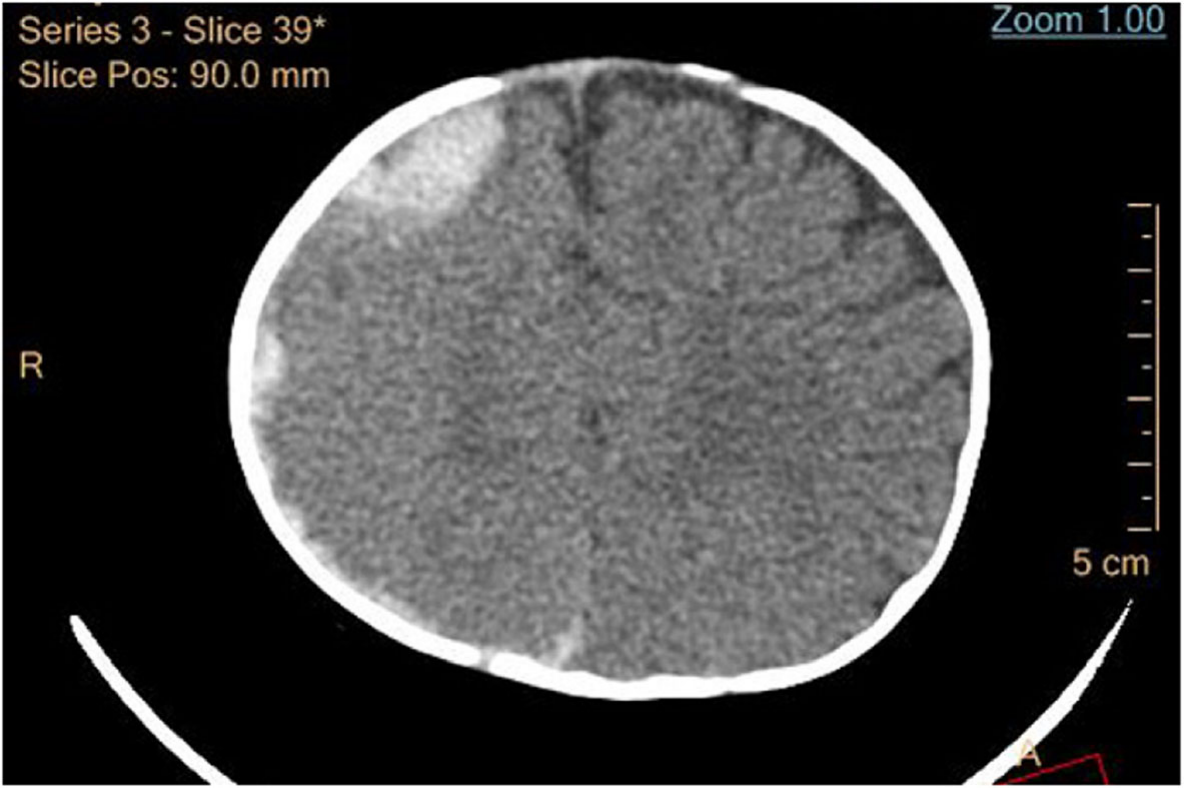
Three months after initial presentation, brain CT scanning showed intracranial hemorrhage
